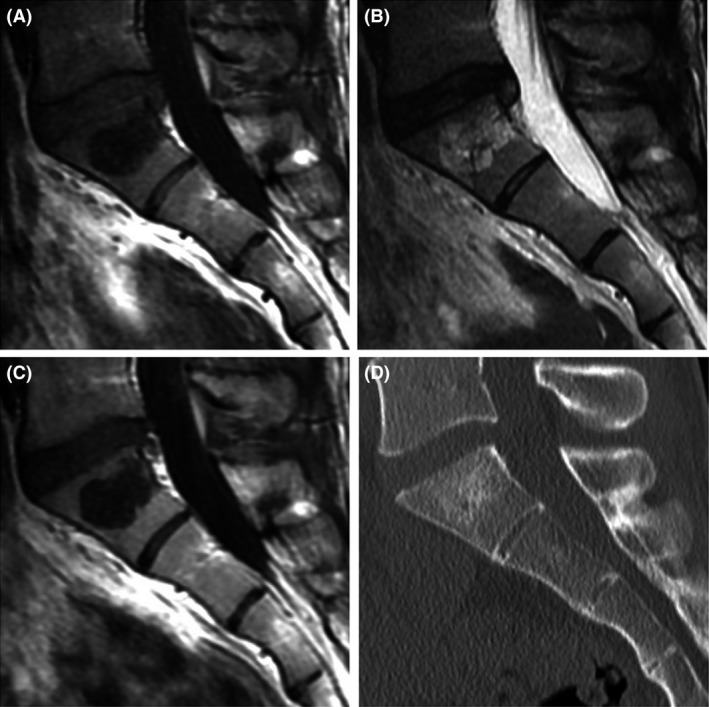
Imaging findings in case 1. On MRI of the sacral spine, the tumorous lesion has clear margins and is enclosed by the S1 vertebral body. The signal intensities are homogeneously low in T1‐weighted images (A) and high in T2‐weighted images (B), and no enhancement is observed in T1‐weighted images with a gadolinium contrast medium (C). No extraosseous lesion or enlargement is observed. CT image demonstrates slight osteosclerosis throughout the tumorous lesion and no osteolytic changes in the vertebral body (D).
